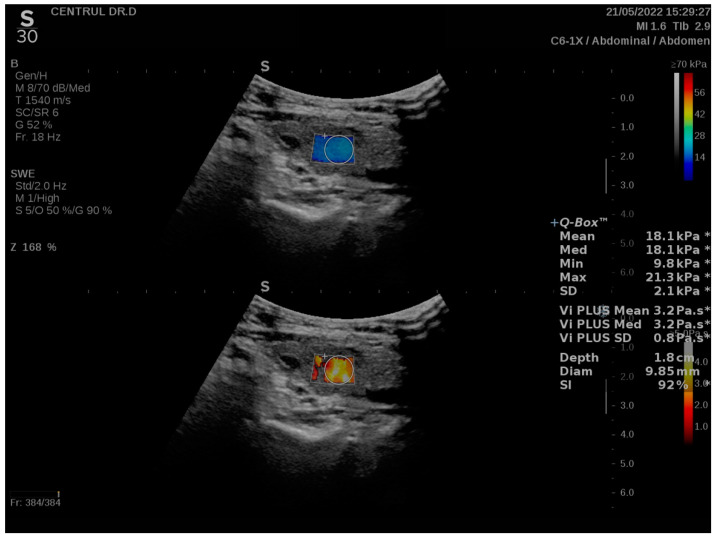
Shear-wave color map (above) and viscosity map (below) overlayed on the greyscale ultrasound image in a case of chronic autoimmune thyroiditis; shear-wave values (mean, median, minimum maximum and standard deviation estimated in kPa), viscosity values (mean, median and standard deviation estimated in Pa·s) and characteristics of the region of interest (depth in cm, diameter in mm and stability index in percentage) are displayed on the right side of the image; the SWE-based parameters are marked with “*”.
